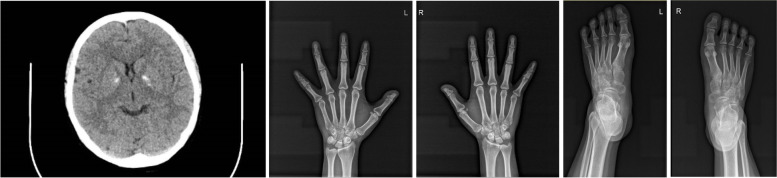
A CT scan of the patient’s brain: Calcification of bilateral globus pallidus (arrows). B X-ray scan of the patient’s hands: no obvious abnormalities were seen (L: left hand, R: right hand). C X-ray scan of patient’s feet: no obvious abnormalities (L: left foot, R: right foot)
